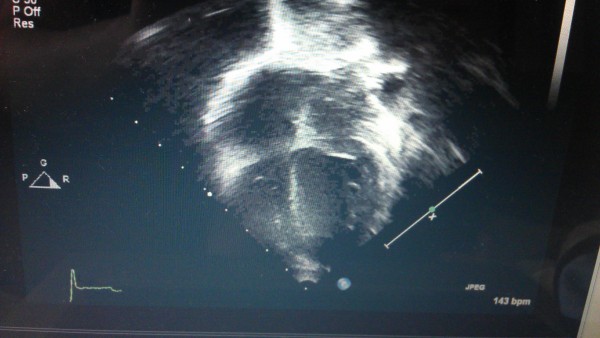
Apparently normal echocardiography after selenium therapy in a 15-month male infant.
